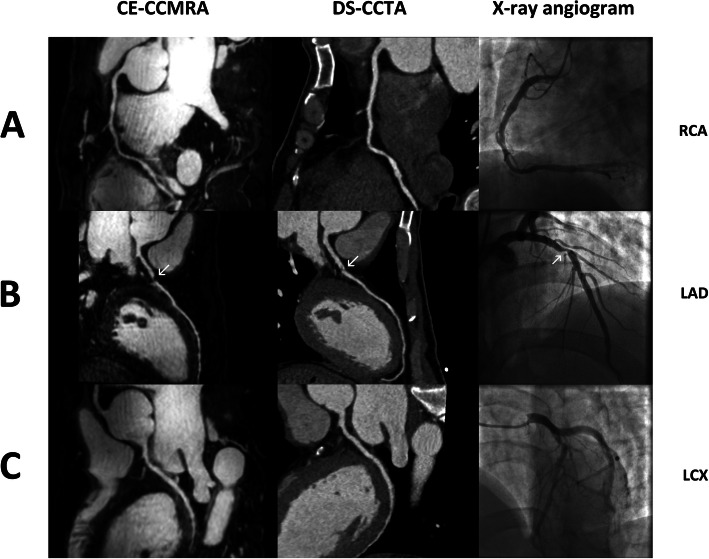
Representative example of CE-CCMRA and DS-CCTA curved planar reconstruction and corresponding x-ray angiography showing a significant stenosis in the proximal LAD (b), normal RCA and LCX (a, c)
